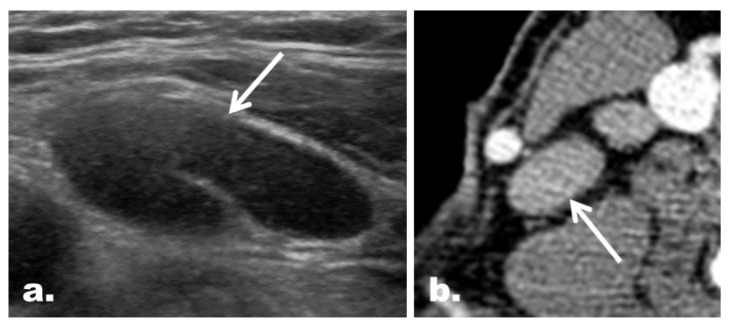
Discordant benign case with the final diagnosis of a benign LN in a 67-year-old woman with PTC. (a) On US, an ovoid LN (arrow) with echogenic hilum was found at the right neck level IV. The LN was classified as probably benign LN on US. (b) On CT, the LN (arrow) was classified as an indeterminate LN because it showed neither central hilar fat nor central hilar vessel enhancement in the absence of any suspicious feature.
